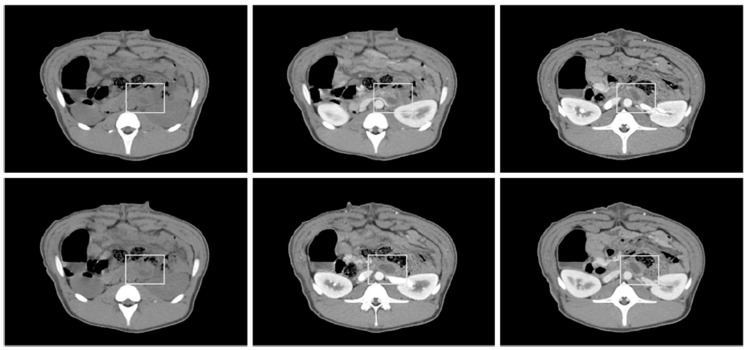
Computed tomography non-contrast (left) image and early (center) and delayed (right) phases of contrast-enhanced CT images: Images showed no evidence of perforation, hemorrhage, ascites, adjacent structural injury, or peri-pancreatic fluid collection. Images showed a solid, low-density lesion in EUS-CILA-treated region (white rectangles).
